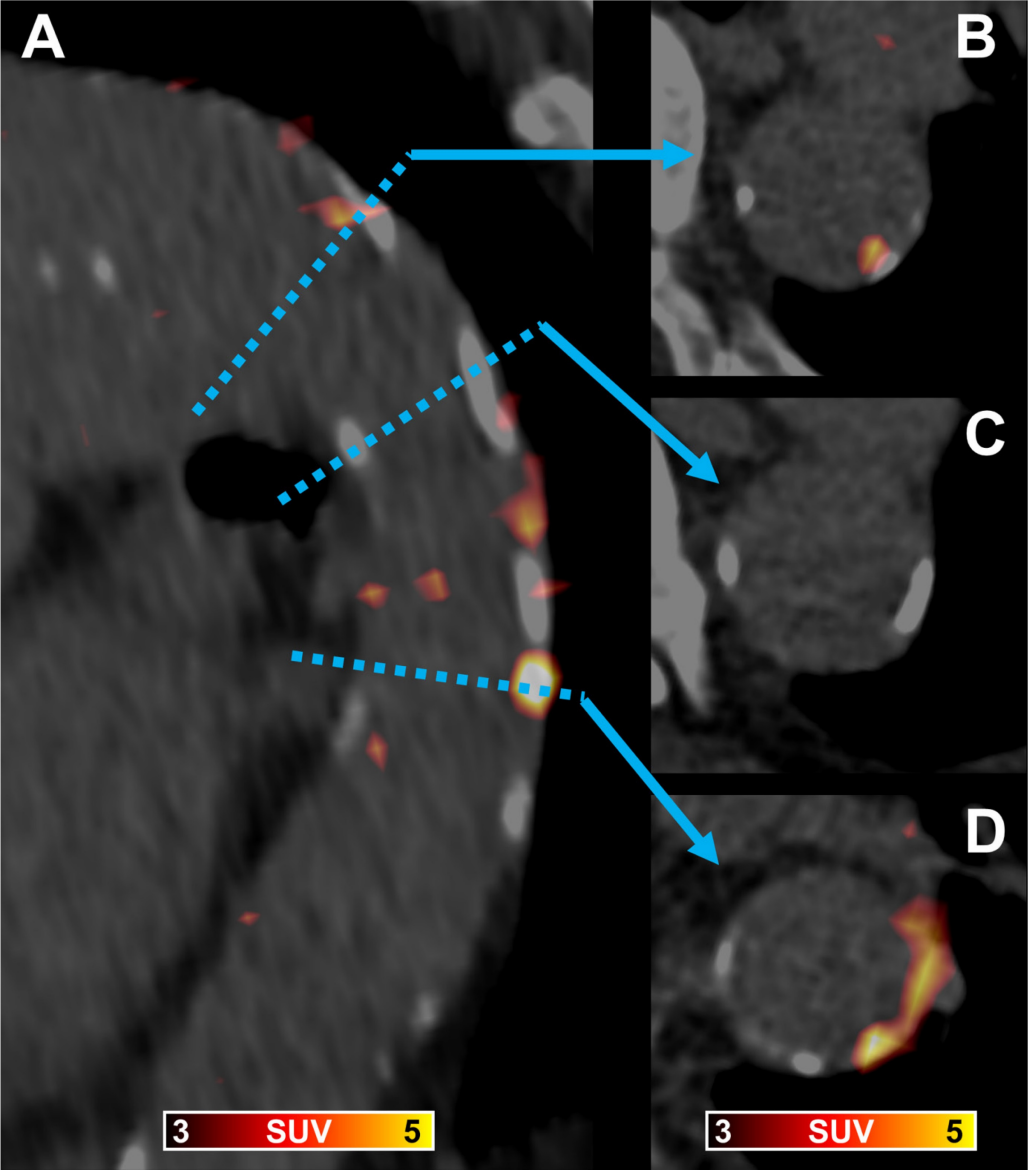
[^68^Ga]Ga-PentixaFor PET/CT in a 89-year old man showing a fused parasagittal image reconstruction of the aortic arch and the descending thoracic aorta (A) and three paraaxial slices (B trough D) at levels indicated by the blue dotted line and arrows. In (B) arterial wall radiotracer uptake is partially co-localized with calcification, in (C) vessel wall calcification without radiotracer uptake is shown, and in (D) vessel wall radiotracer uptake without corresponding calcification and calcification without co-localized radiotracer uptake is visible.
